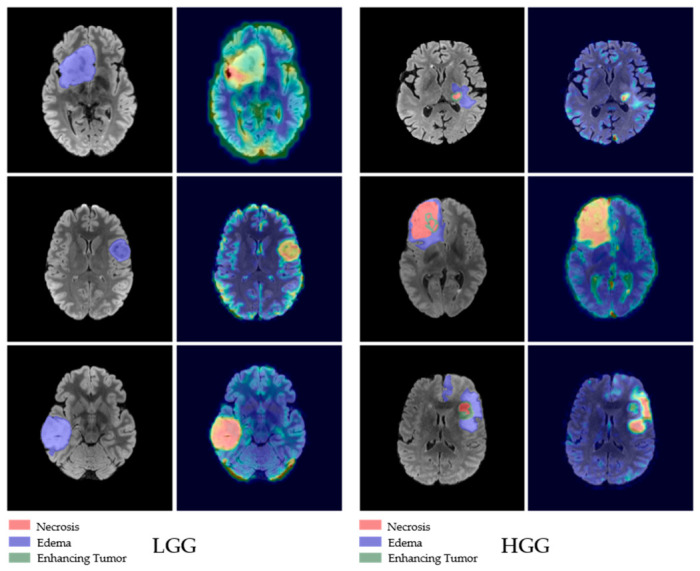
Qualitative visualization of three LGG and three HGG validation cases from the UCSF-PDGM dataset. For each category ground-truth tumor masks (left) are shown alongside the corresponding Grad-CAM activation maps (right) overlaid on the original axial MRI slices.
